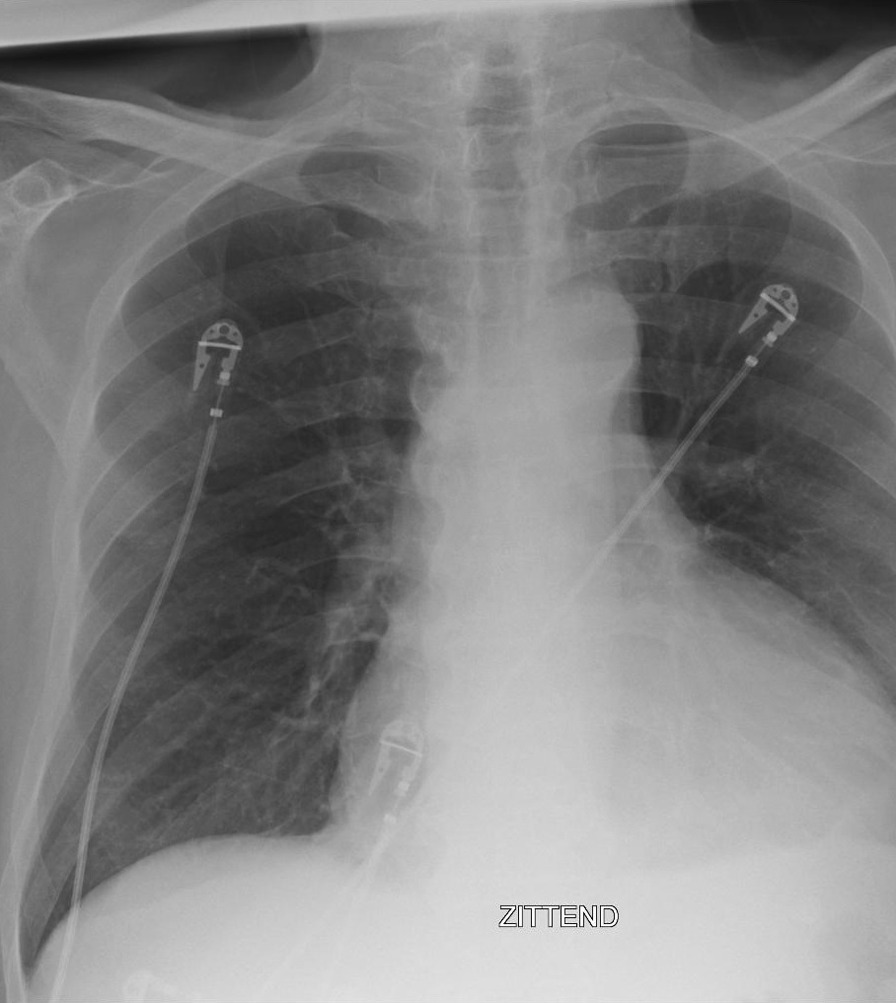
Chest X-ray showing an enlarged cardiac silhouette and left lower-lobe atelectasis with mild volume loss. The Dutch text in the X-ray image means “sitting”
